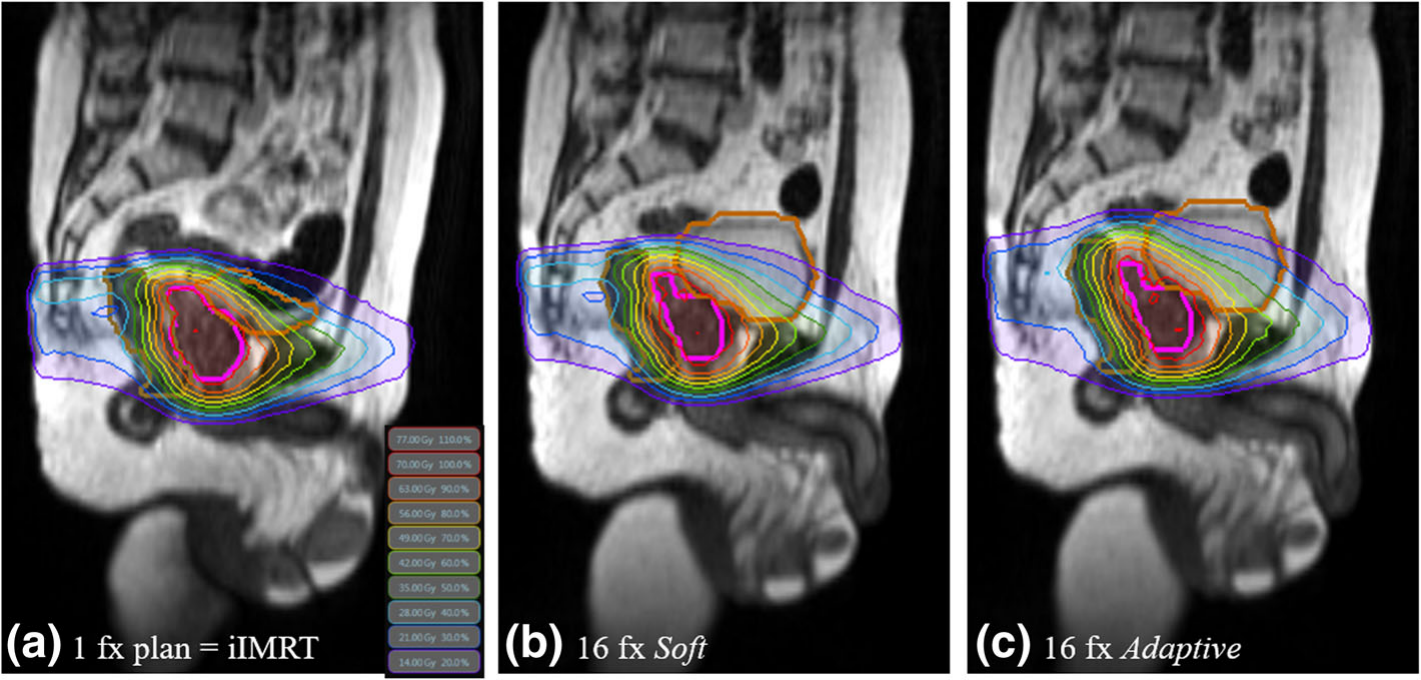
Representative dose distributions with three plans: (a) initial, (b) *Soft*, and (c) *Adaptive*. All plans excluding the initial plan had the same organ structures and MRI
